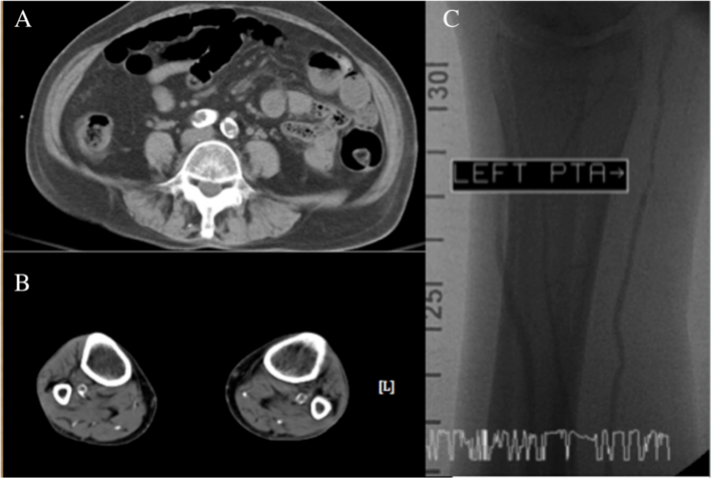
Diffuse calcifications and atherosclerotic changes of the vessels. Computed tomography angiographies without enhancement showed (A) marked vascular wall calcifications and atherosclerotic changes of bilateral common iliac arteries and (B) marked calcification of the large and small arteries of both lower legs. (C) Conventional angiography showed a 70% stenosis in left posterior tibial artery, indicating atherosclerotic change of the vessel.
